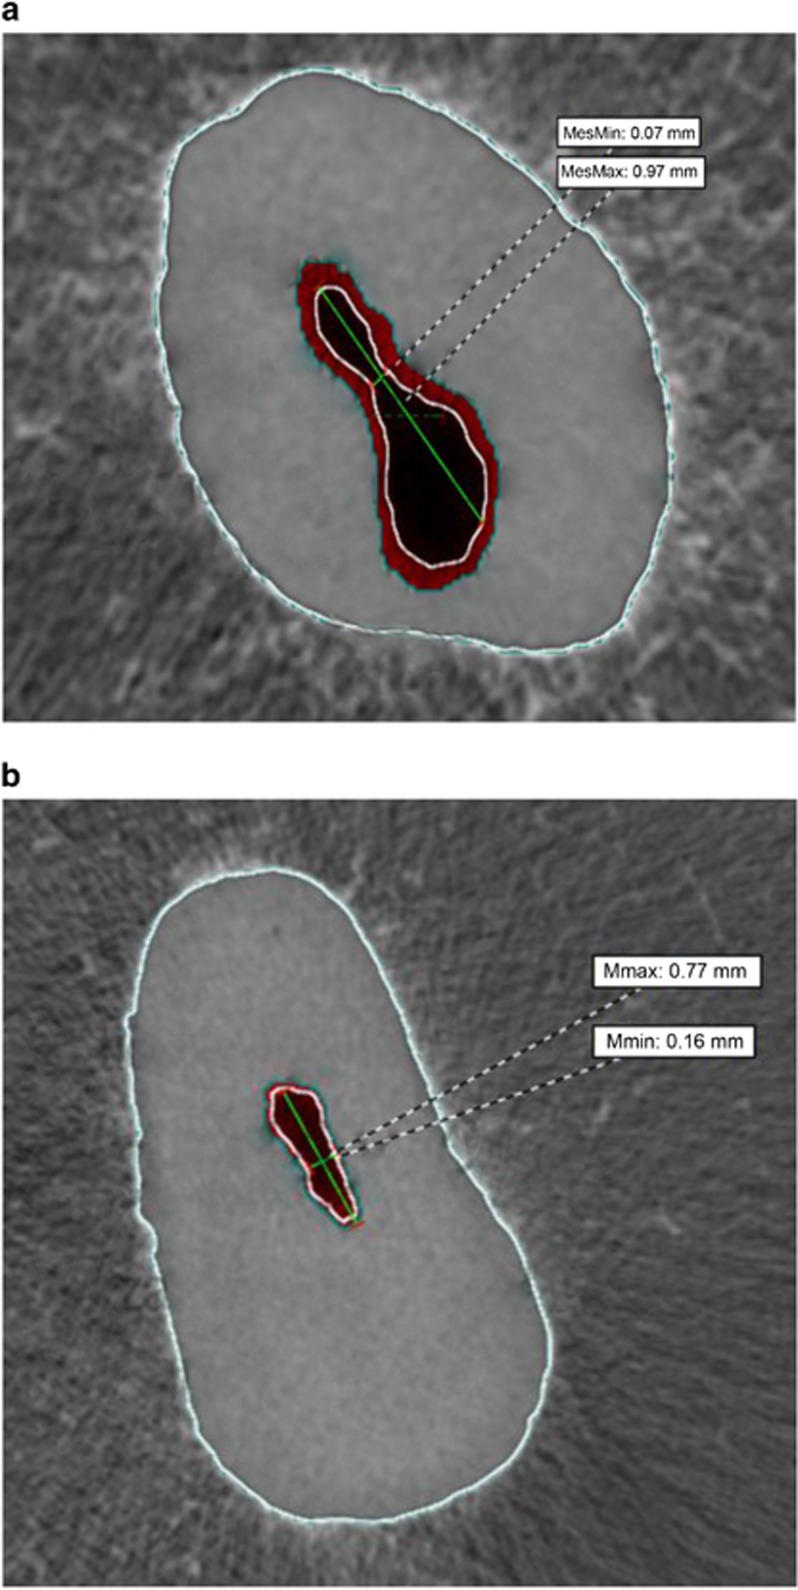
Micro-computed tomography horizontal plane at the physiological foramen level of the distal and palatal roots of a mandibular (a) and maxillary molar (b), respectively. In this plane the oval shape of the foramina can be clearly distinguished. The differences between the major and minor diameters of the foramina denote the demanding challenges to achieve a clinically ideal preparation of the foramen. max, maximum; min, minimum.
